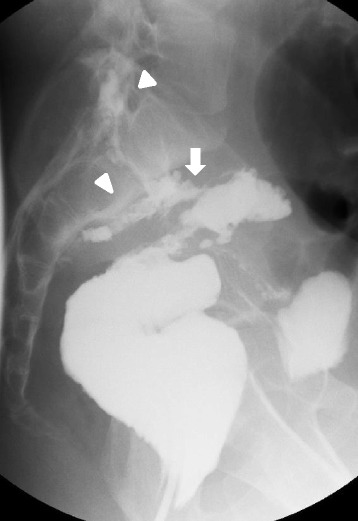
Gastrografin radiographic examination shows leakage from the top of the ileal pouch (*white arrow*) to the spinal canal at the L5–S1 level, forming a spinal epidural abscess (*white arrowheads*)
